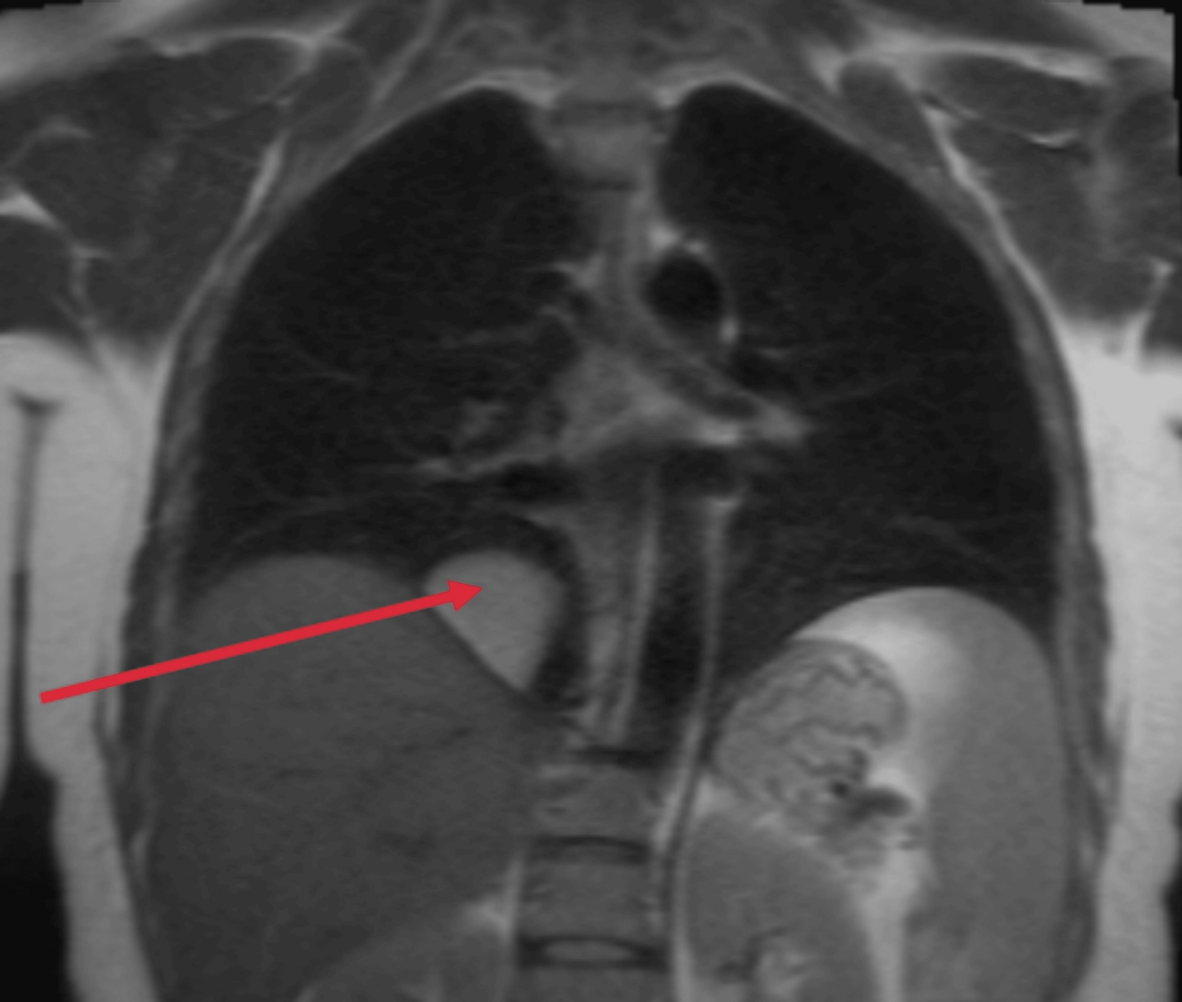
Coronal view of the cardiac MRI. The red arrow points to the pericardial cyst.
